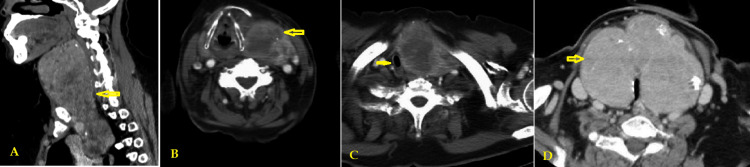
Computed tomography scan showing retrosternal thyroid mass (A) lateral view (arrow), (B) transverse section (arrow), (C) mass causing tracheal deviation (arrow), (D) big thyroid mass with calcifications (arrow).
